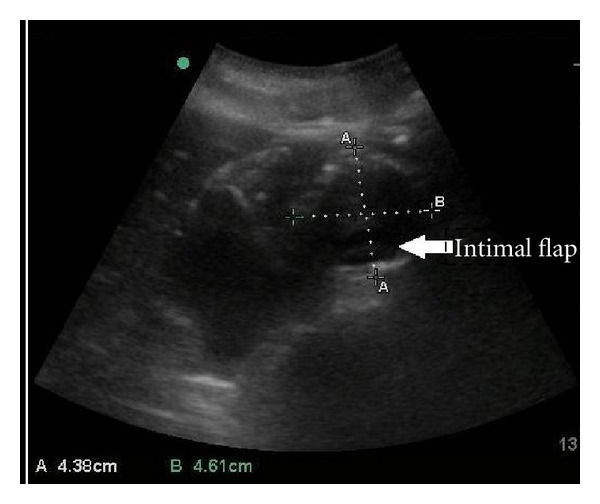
Abdominal ultrasonography demonstrating intimal flap (arrow).
